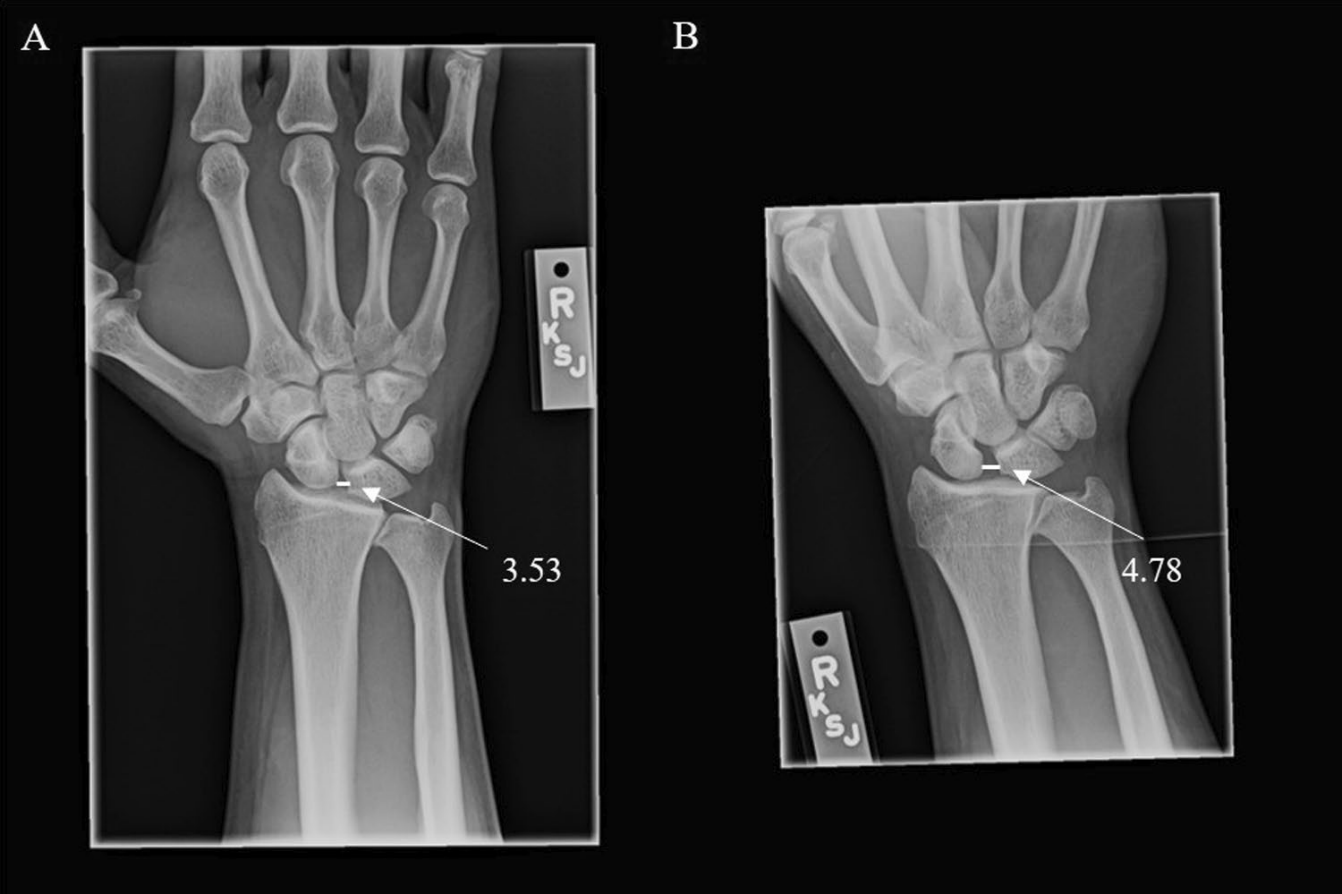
Radiograph of PA (A) and tangential Moneim view (B) with average SL gaps of 3.53 mm and 4.78 mm at 12 years from injury. See radiocarpal degenerative changes, confirmed at surgery
